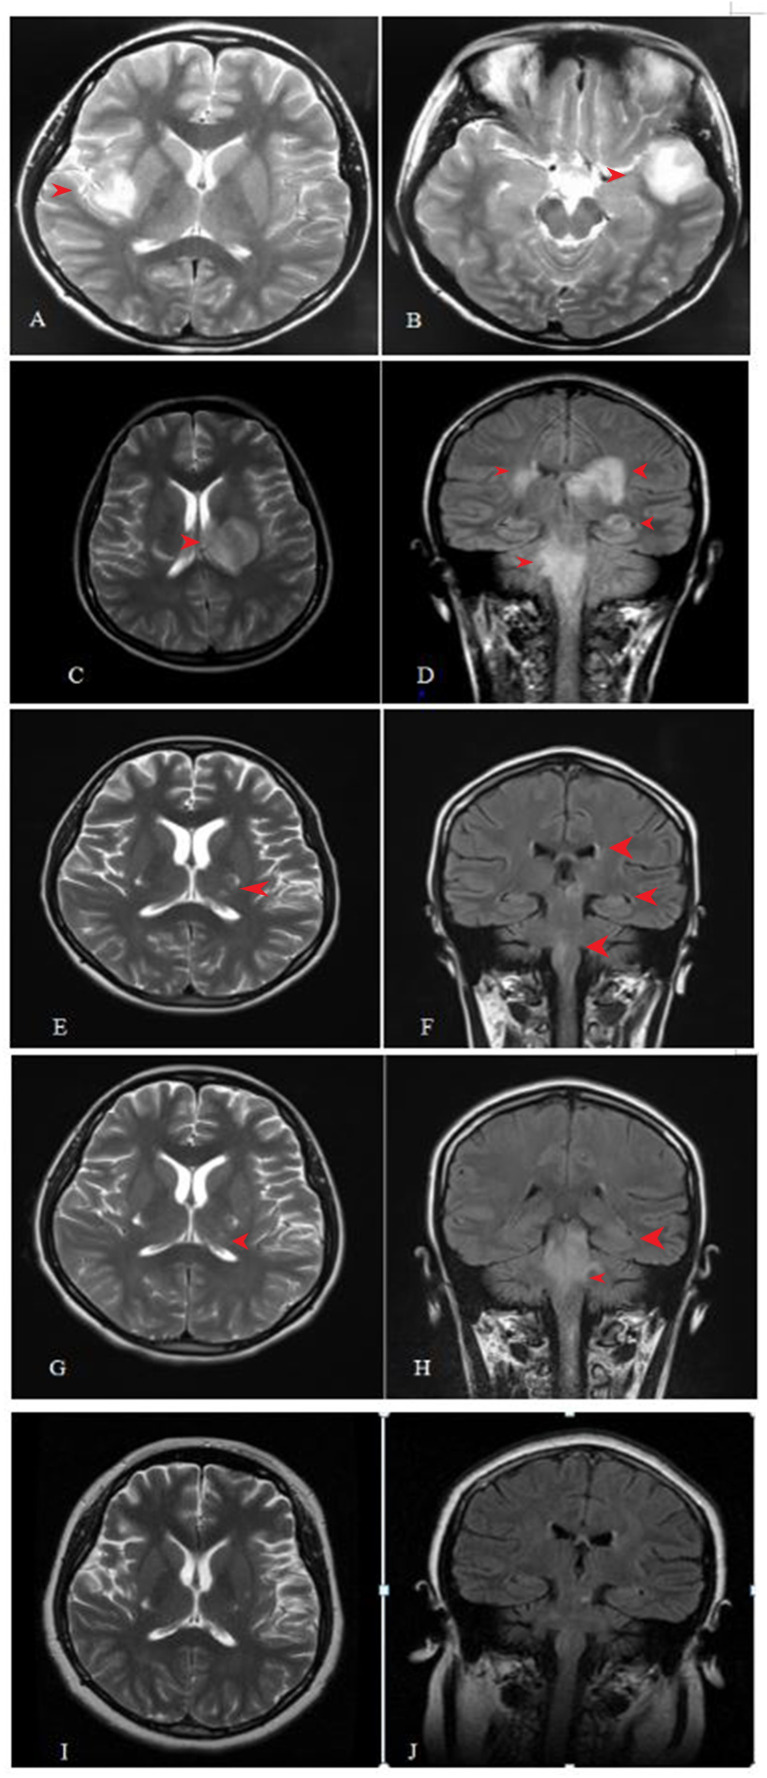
Cranial magnetic resonance imaging (MRI) examination during the process of disease. (A, B) lesions in left temporal lobe and right insular lob. (C, D) lesions in the medulla, pons, the right cerebral peduncle, bilateral posterior limb of internal capsule, bilateral Insula medialis, left temporal lobe, and right posterior horn of lateral ventricle. (E, F) lesion was significantly smaller than before in 7 days after abortion. (G, H) Some new demyelinating-like lesion was found in 26 days after abortion. (I, J) Lesions in brain stem, bilateral basal ganglia had disappeared which was observed by brain MRI scan at September 8, 2017 during following-up.
